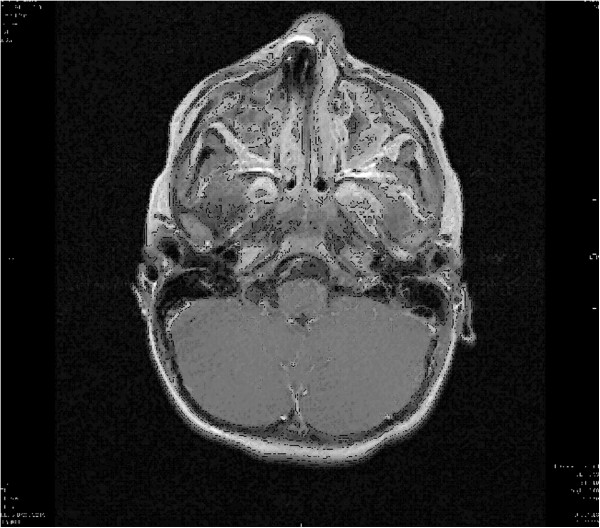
Magnetic resonance image of the head
